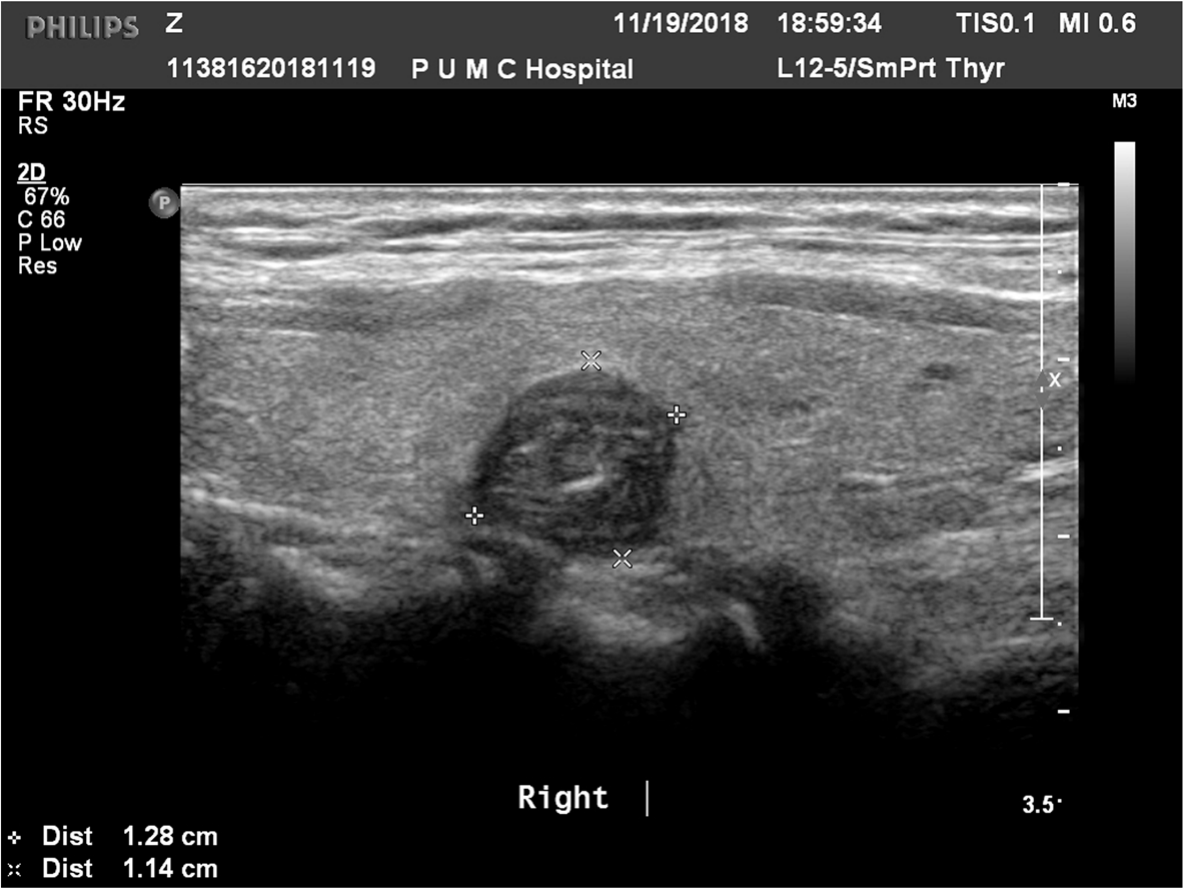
Ultrasonography of the parathyroid carcinoma
